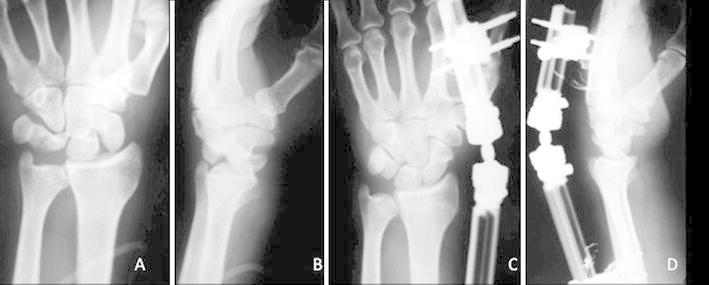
Posteroanterior (a) and lateral (b) radiographic views of the left wrist of a 59-years-old male with a perilunate dislocation. Posteroanterior (c) and lateral (d) lateral views of the wrist joint before the application of K-wires, showing successful restoration of normal carpal alignment, which was achieved via ligamentotaxis using a wrist external fixation
